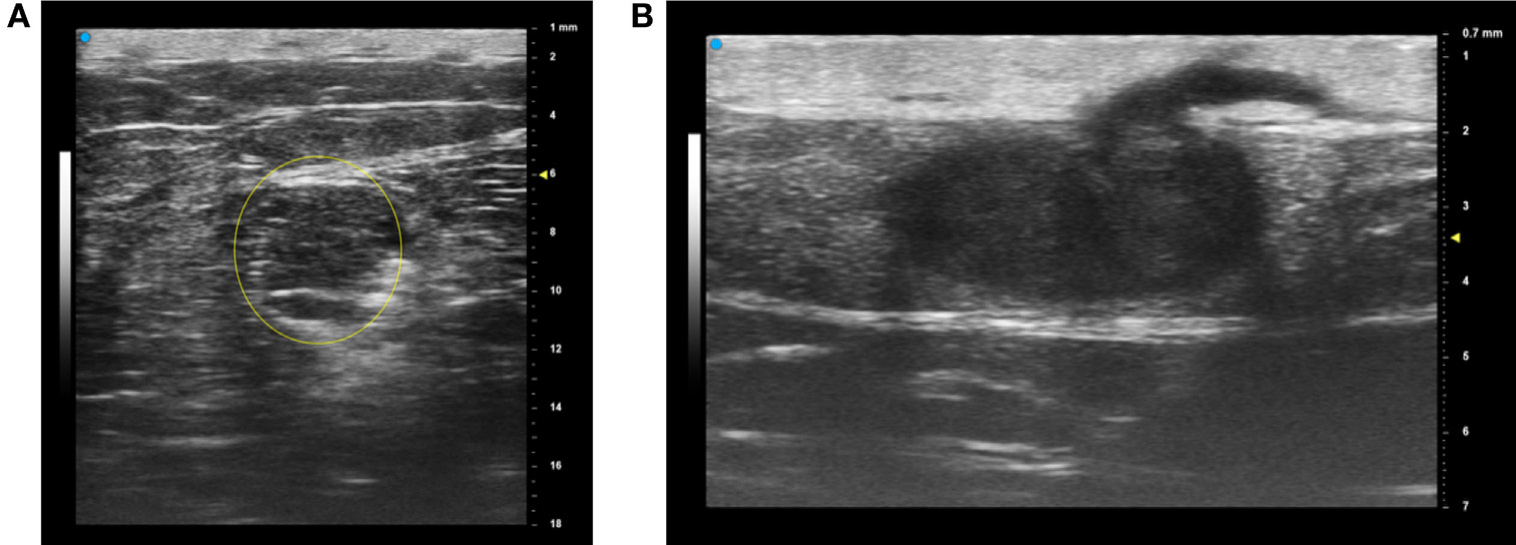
The most recent array-based ultrasound technologies for dermatology provide a choice of operating frequency, which enables the operator to “trade off” the field of view and resolution. For example, a deeper lesion (lipoma) is best imaged with a lower center frequency of 30 MHz (A) while a more superficial lesion and hair are better imaged at a center frequency of 50 MHz (B) with higher resolution but a smaller overall field of view. These images are courtesy of VisualSonics. Permissions obtained to reprint from Vevo MD for Dermatology (27), retrieved from https://www.visualsonics.com/product/imaging-systems/vevo-md Copyright 2017 Fujifilm VisualSonics Inc.
